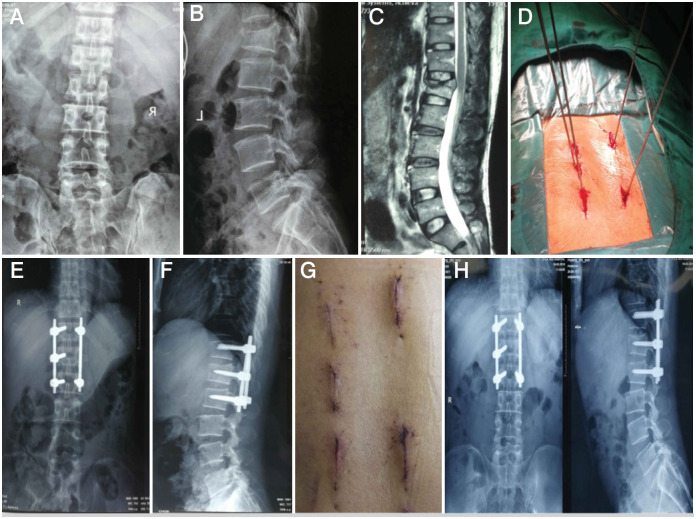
A 32-year-old man with a burst fracture of the lumbar vertebrae. A, B: preoperative X-ray; C: preoperative MRI; D: intraoperative positioning and then inserting the guide needle; E, F: postoperative X-ray; G: postoperative incision; H: review of X-ray after 1 year of operation.
